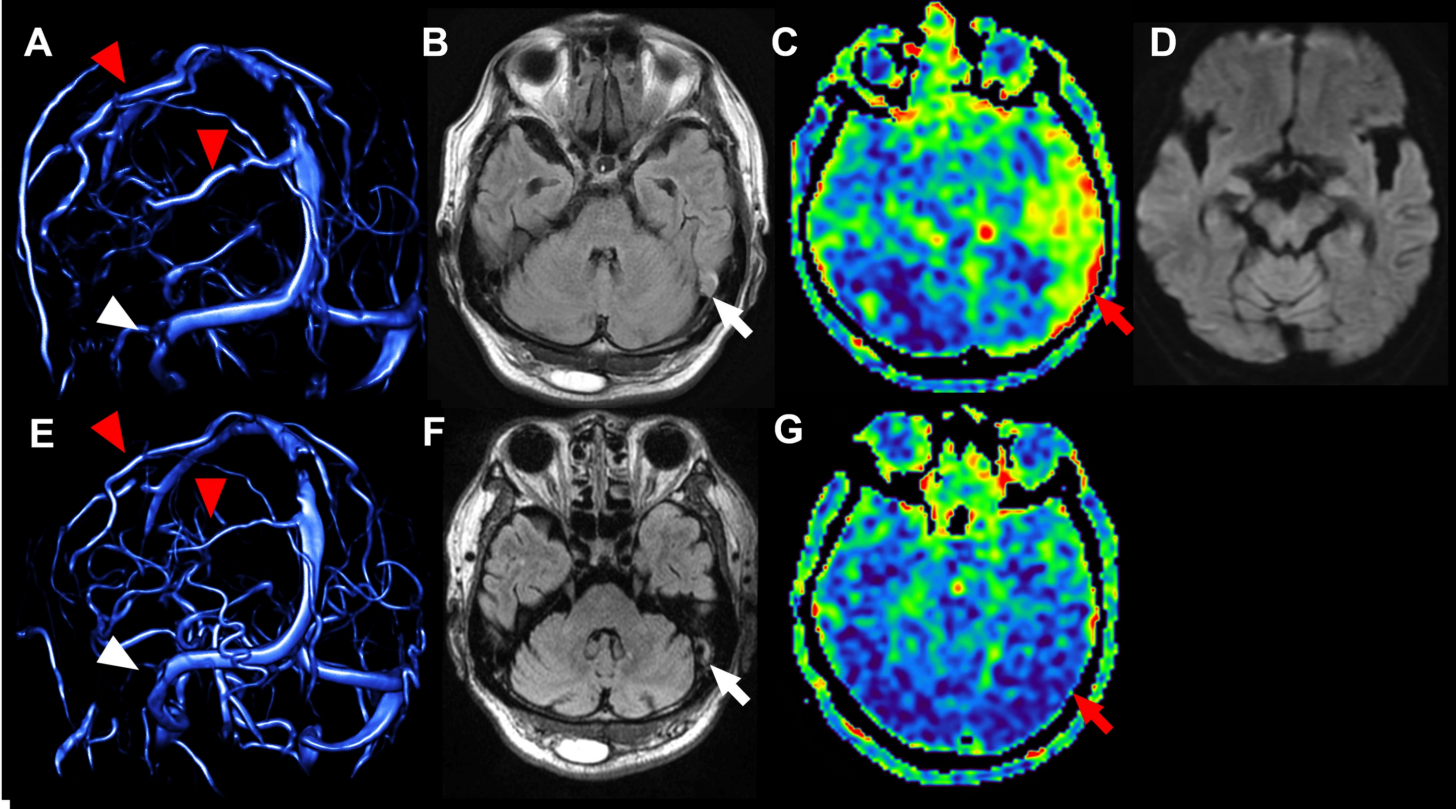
The transitions in the occlusion site of venous sinus and cerebral blood flow. (A) Magnetic resonance venography (MRV) on admission (day 0). The white arrowhead indicates the occluded portion of the left sigmoid sinus. The red arrowheads indicate dilated cortical vein. (B) Fluid-attenuated inversion recovery (FLAIR) on day 0. The left sigmoid sinus was nearly occluded. No significant differences in signal values were observed in the bilateral temporal lobe. (C) Arterial spin labeling (ASL) of day 0. The red arrow indicates increased cerebral blood flow (CBF) in the left temporal lobe. (D) The diffusion-weighted image of day 0 showed no ischemic or hemorrhagic lesion in the temporal lobe. (E) MRV on day two. The white arrowhead indicates the recanalized left sigmoid sinus. The red arrowheads indicate cortical veins that no longer demonstrate lateral asymmetry. (F) FLAIR on day two. The thrombus in the left sigmoid sinus has shrunk, and the stenosis has improved slightly. No laterality of signal intensity was observed in the bilateral temporal lobe. (G) The red arrow indicates that CBF in the left temporal lobe changed symmetrically.
